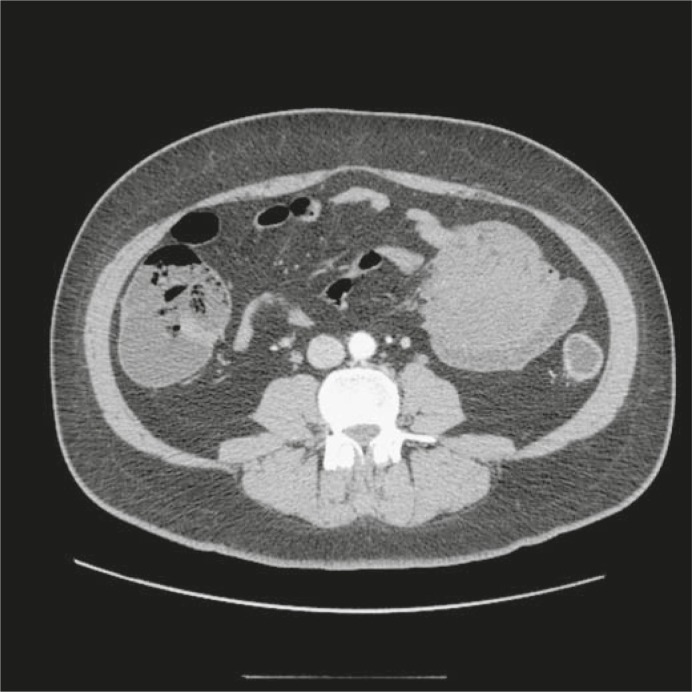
CT scan shows the desmoid tumour of the mesentery infiltrating the small bowel: a well-defined hypodense and homogenous mass diffusely attached to the bowel wall.
